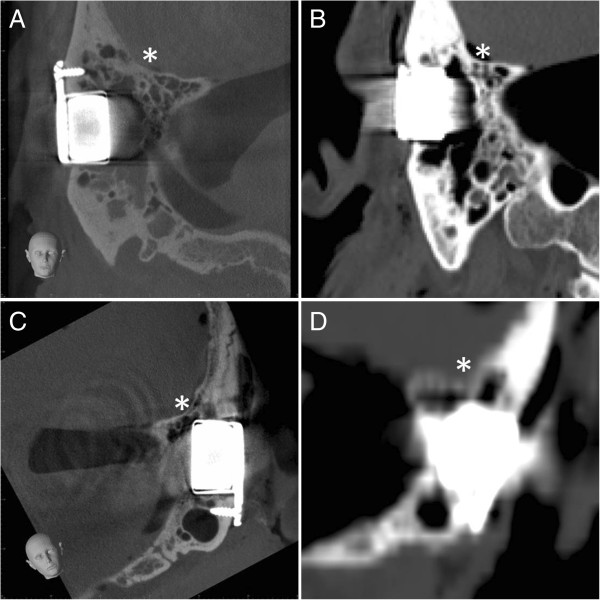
Positional relation of the implant to the posterior cranial fossa. Visualisation of the implants in CBCT (A and C) and in CT (B and D) for both prepared skulls (A and B, head 1; C and D, head 2) in relation to the surrounding brain structures (*).
